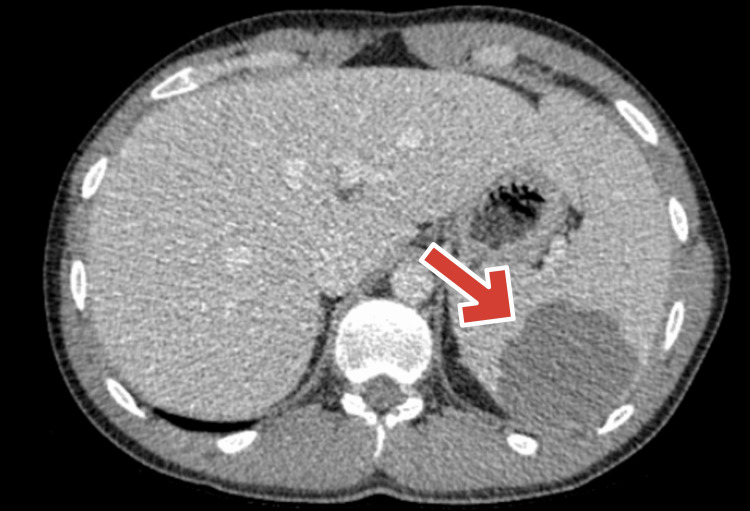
Contrast-enhanced CT abdomen/pelvis (pre-op day 1) showing a 5.6x3.8 cm posterior splenic lesion with hepatosplenomegaly. Arrow pointing at the splenic lesion.
